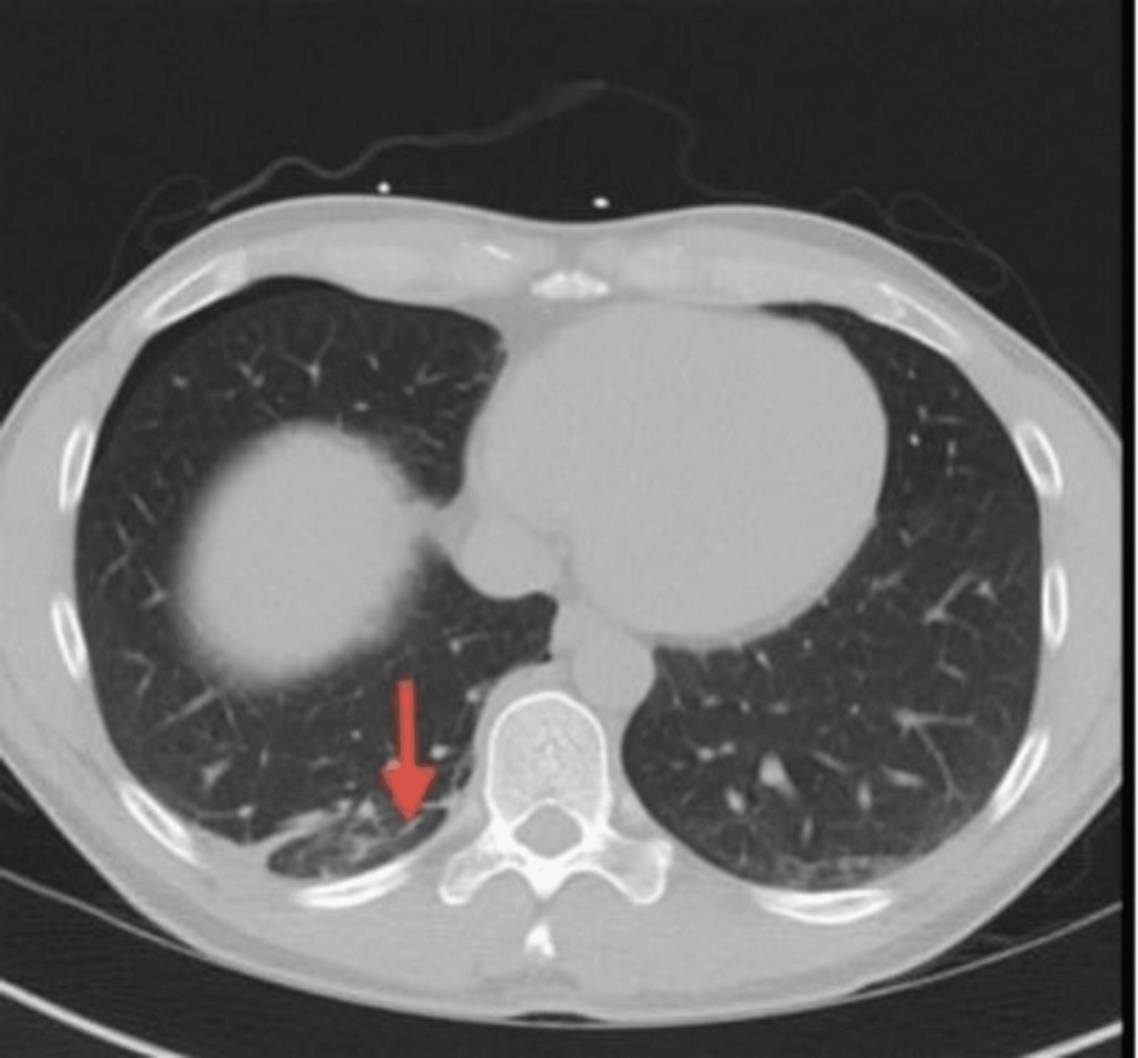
CT scan with arrow pointing to the retained injection needle (axial view)
